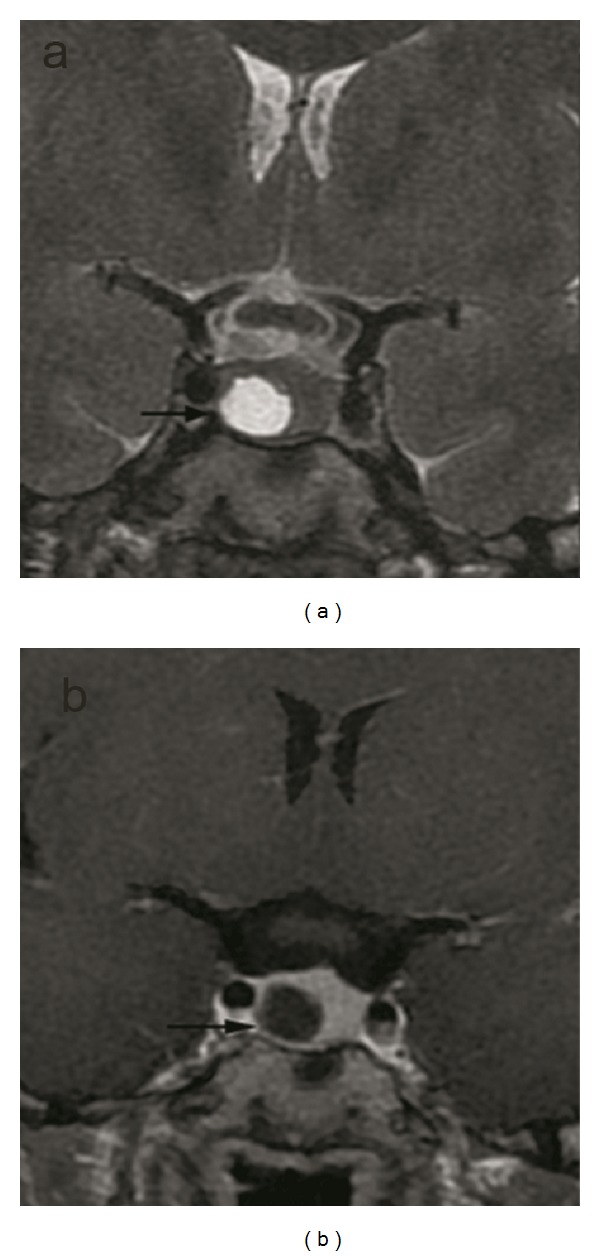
Rathke's cleft cyst or cystic macroadenoma with hyperintense signal intensity on the T2-weighted coronal image (a) (arrows) and hyperintense signal intensity on the T1-weighted coronal image enhanced with IV-GBCM (b) (arrows) are shown. Diffuse enlargement of the gland and the invasion of the right cavernous sinus can be demonstrated on both images.
